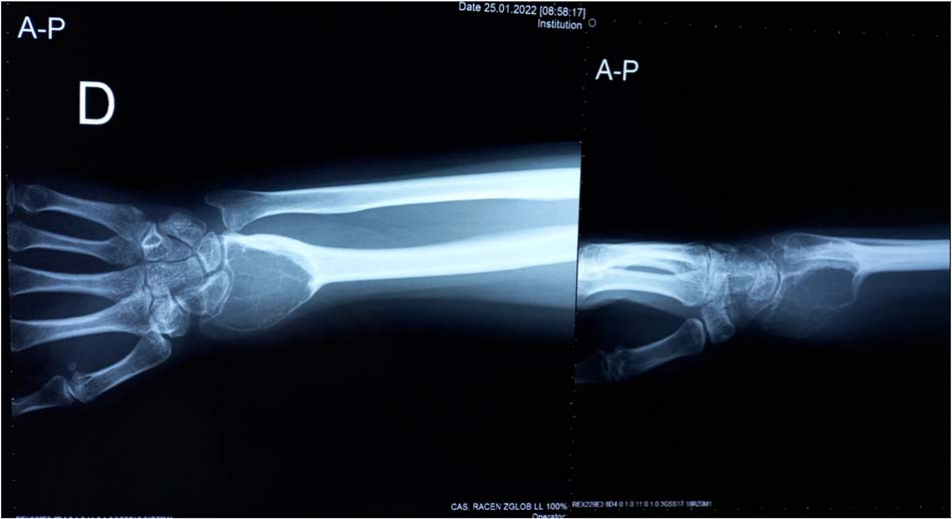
Plane anterior–posterior X-ray of the right forearm.
